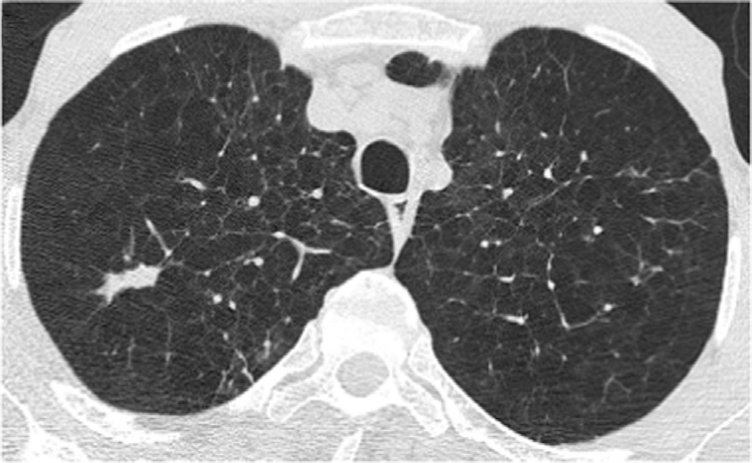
CT thorax from December 2015 showing a spiculated elongated nodule in the right upper lobe.
